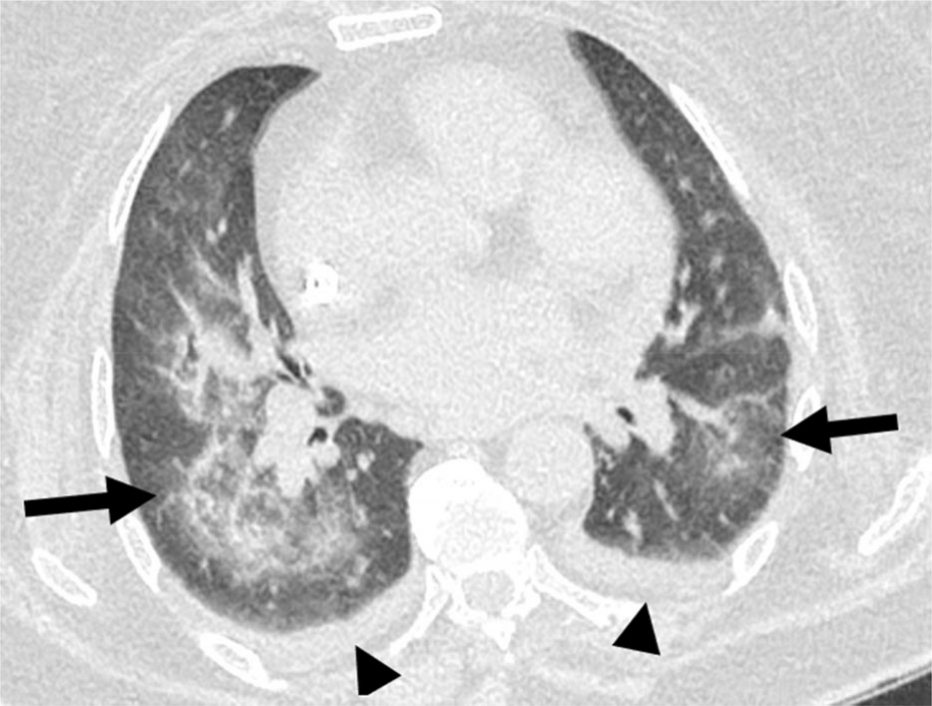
Non‐contrast axial CT chest of a 71‐year‐old female patient with End‐Stage Renal Failure secondary to GPA showing ground‐glass and air space densities throughout both lungs (arrows), which may represent diffuse alveolar haemorrhage in the setting of vasculitis. Small bilateral pleural effusions are also present (arrowheads).
